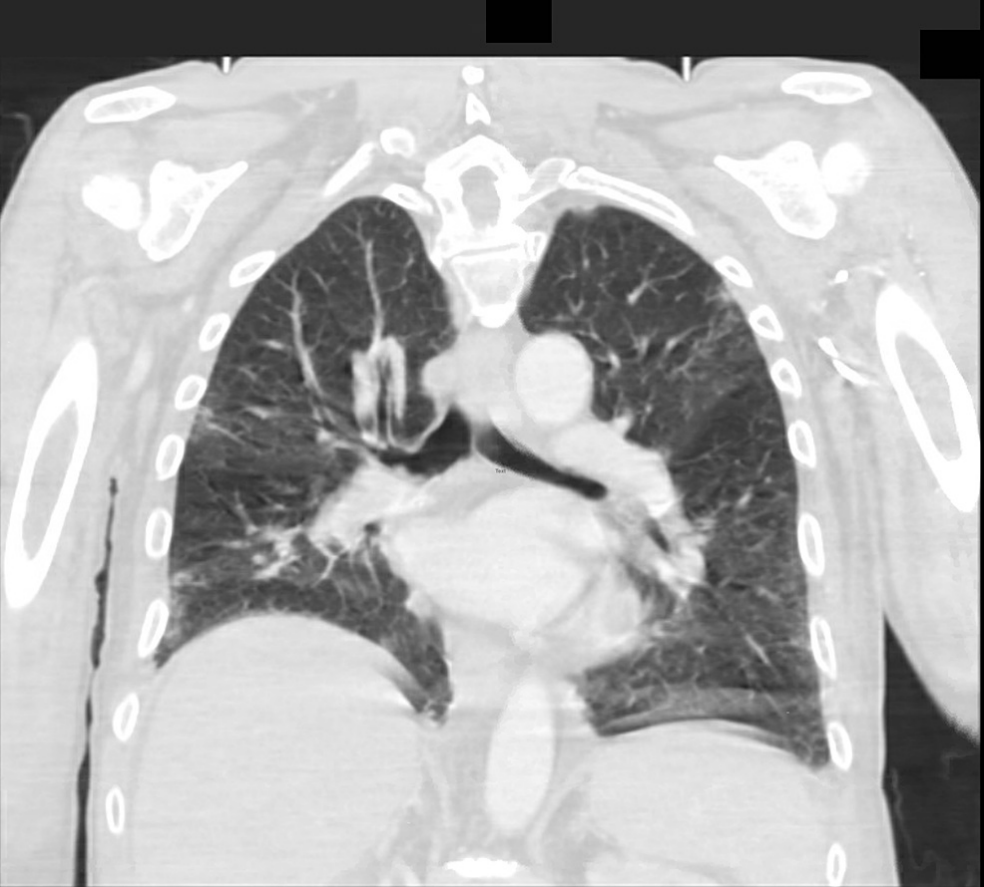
CT of the chest: coronal view CT: computed tomography
